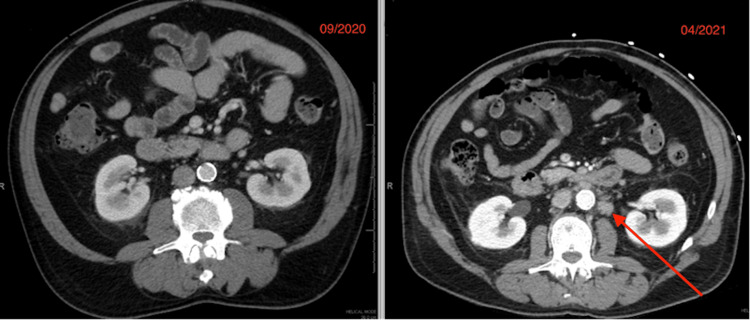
CT Abdomen and pelvis lymphadenopathy in comparison to prior imaging
